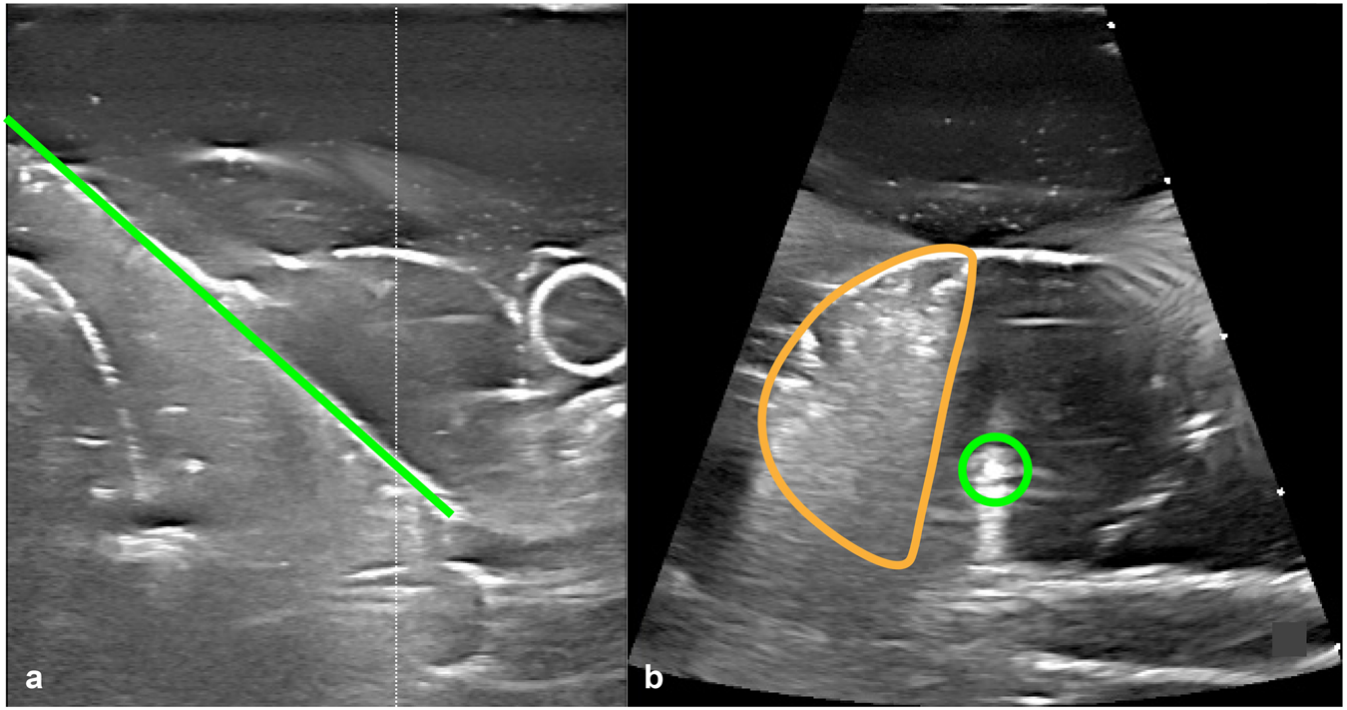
An example of the use of dual-plane imaging (three-dimensional ultrasound), the electrode (green) was placed using both planes (a, b) allowing for easy placement with respect to the ablation boundaries (orange) and nodule edge
